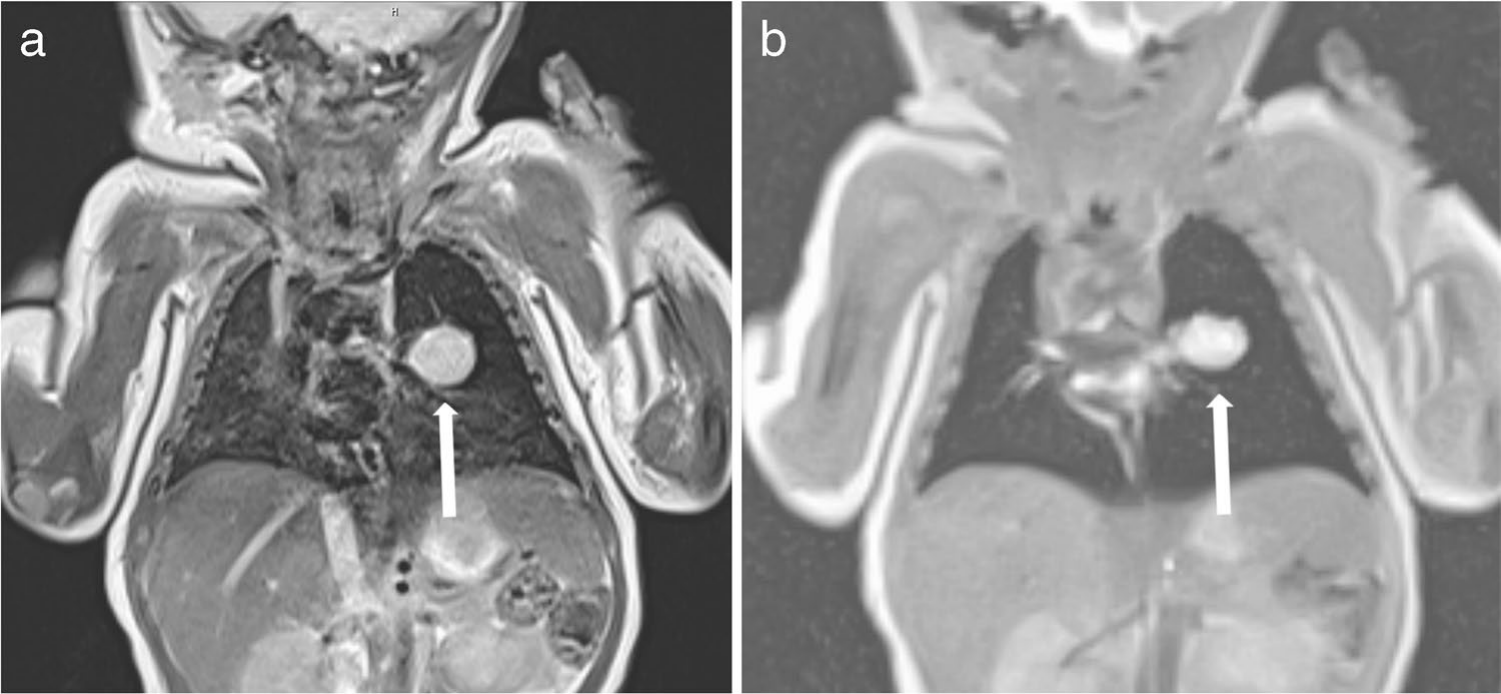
Contrast appearance of the T2-weighted volume coverage sequence in a 2-day-old girl with a bronchocele *(arrows*) of the left upper lobe bronchus and hyperinflation of the entire left upper lobe in coronal projection. The hyperinflation of the upper lobe was recognizable only to a limited extent on the T2 sequences. a An image of the bronchocele on the T2-turbo spin-echo (TSE) standard sequence. b An image of the real-time T2-volume coverage sequence. The acquisition time of the triggered T2 TSE sequence was 3:50 min; the T2-volume coverage acquisition time was 12 s in free breathing “(corresponding video: Online Supplementary Material 2)
